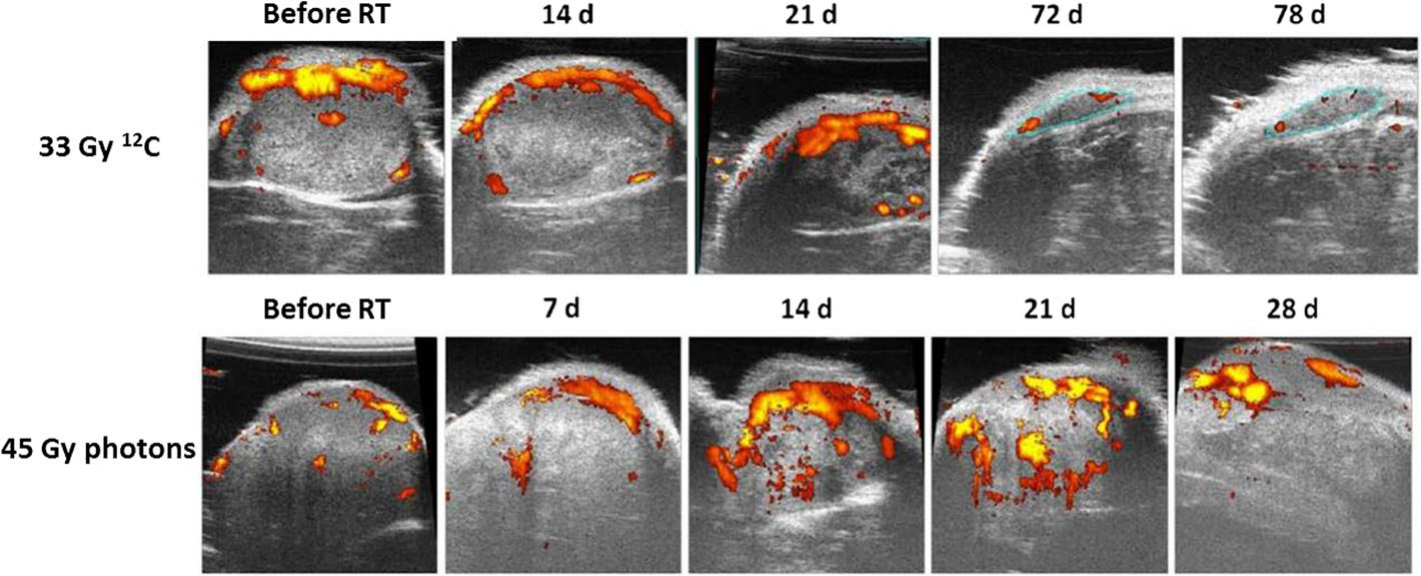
Representative axial images of color-coded power doppler ultrasound overlayed with a B-Mode image showing a middle HI-tumor section before and after selected time points after single doses: yellow and red pixels represent the blood flow. The blue contours after 72 and 78 days in the upper row represent the persisting residual tumor volume of a local controlled HI-tumor. The lower panel describes the time course of a photon treated HI-tumor without local tumor control / with a regrowing tumor showing continuous volume and blood flow increase. After carbon ion irradiation, the tumor volume reduction as well as the decrease in power doppler signal reveals a tremendous decrease in blood flow which is not seen after photon treatment at early time points
